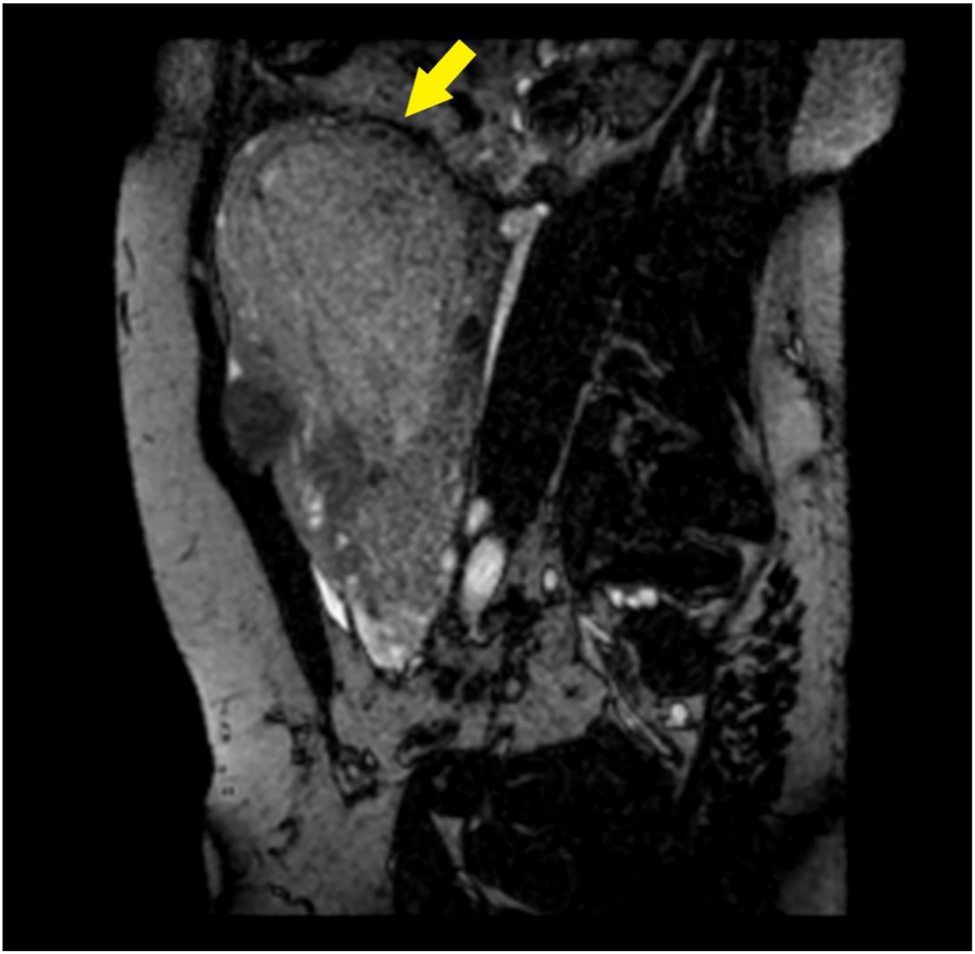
Contrast-enhanced MRI. Para-sagittal BFFE acquisition: the placenta causes marked thinning of the myometrium (arrow).
